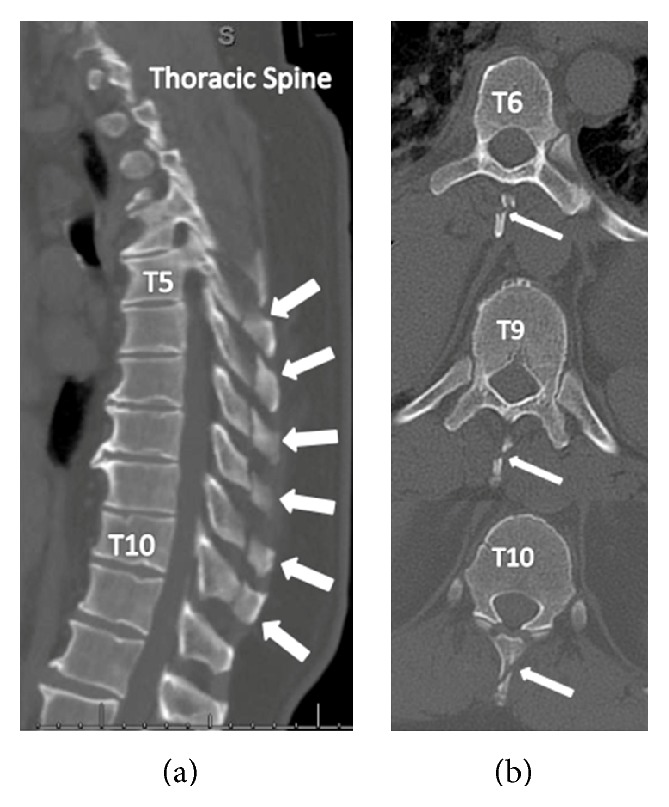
(a) Sagittal computed tomography (CT) image demonstrating thoracic spinous process fractures (arrows) spanning from T5 to T10. (b) Axial CT showing select (T6, T9, and T10) spinous process fractures (arrows). No evidence of vertebral body or intralaminar extension is noted.
